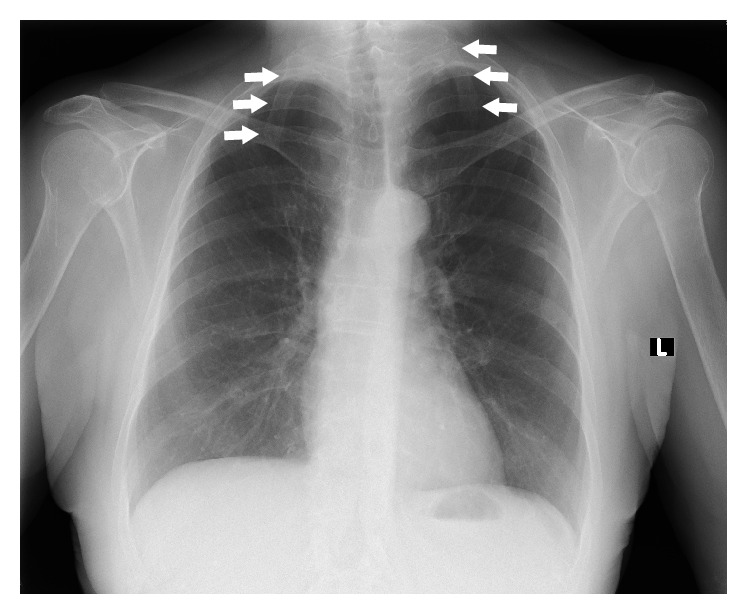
Chest radiograph showing bilateral cervical ribs (arrows) attached to the seventh cervical vertebra.
